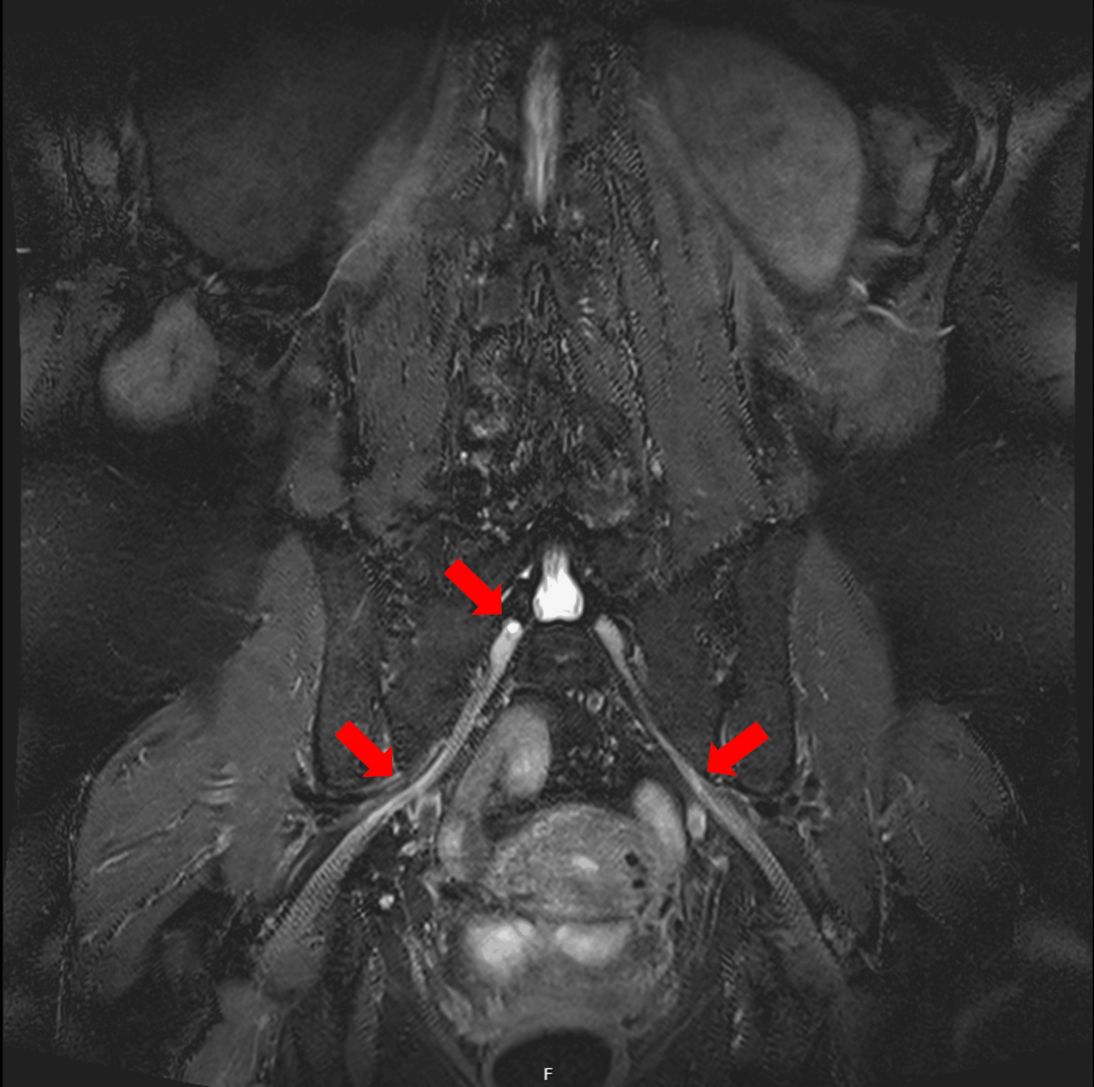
T2-weighted short tau inversion recovery (STIR) hyperintense signal highlighted with red arrows in bilateral lumbosacral plexus on coronal magnetic resonance imaging.
